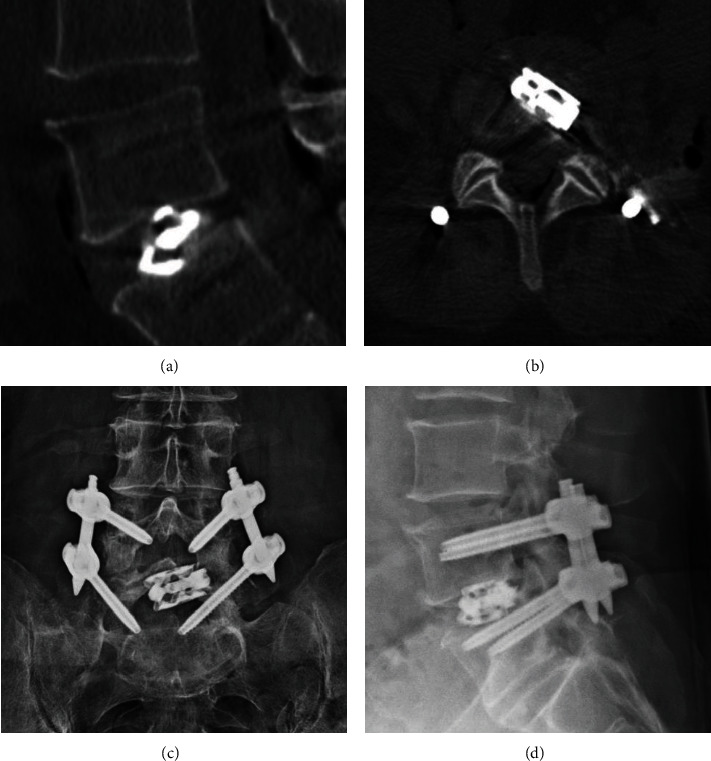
Representative radiographic outcomes of full-endoscopic TLIF. (a) Sagittal CT slice obtained on the day of surgery confirmed adequate graft positioning and L4-5. The expanded cage resulted in disc space recovery, as intended. (b) Associated axial view of implanted hardware revealed expected oblique graft orientation. Procedure-related free air in the retroperitoneum was also noted. (c) Plain anterior-posterior films obtained at short-term follow-up affirmed hardware placement. (d) Associated lateral X-ray also did not reveal evidence of hardware complication.
